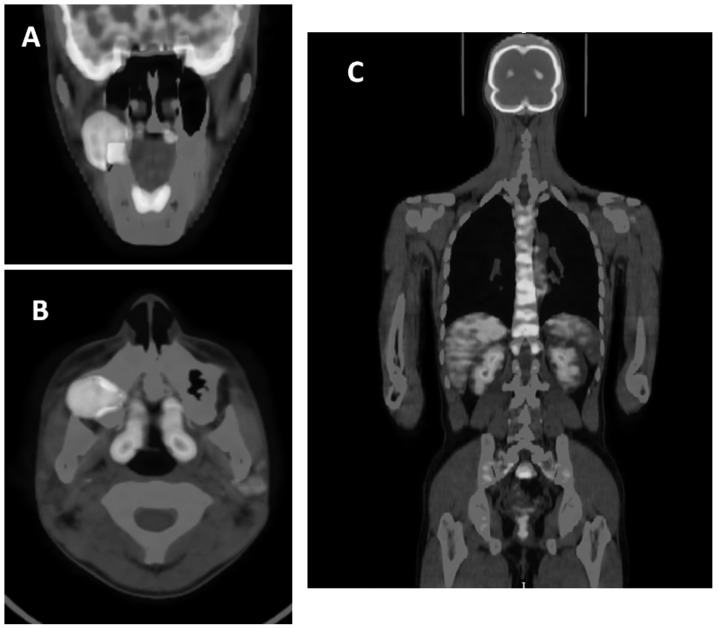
FDG-PET images. (A) axial and (B) coronal FDG-PET/CT images revealing a slight FDG uptake in the primary tumor of the right maxilla and bilateral superior internal jugular nodes. (C) No abnormal uptake, which would indicate distant metastasis, was observed on FDG-PET images. FDG-PET, ^18^F-fluorodeoxyglucose-positron emission tomography; CT, computed tomography.
